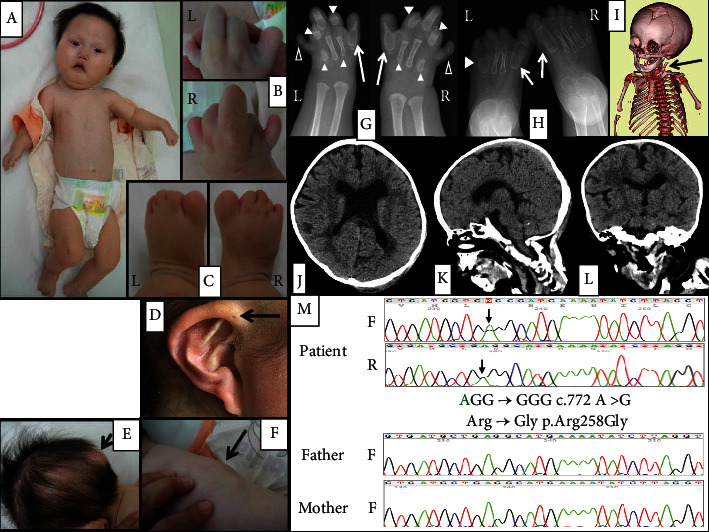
Clinical features, radiological examination findings, and genetic analysis results of our patient. (a–f) Clinical features of our patient at the first visit to our hospital. Left (L); right (R). (a) General appearance. Thin eyebrows, low-set ears, hypoplastic mandible, narrow chest, and shallow umbilicus were observed. (b) Hands. Incomplete syndactyly was observed in both hands. All fingers lacked nails. (c) Feet. Incomplete syndactyly was observed in both feet. All toes lacked nails. (d) Right ear. The lower part of the ear was hypoplastic. A periauricular sinus (arrow) was observed. (e) Flare-up observed at the occipital region of her head (arrow). The hair was sparse. (f) Flare-up observed at her back (arrow). (g–l) Results of radiological examinations. Left (L); right (R). (g) Roentgenograph of the hands at 7 months of age. Agenesis of all distal phalanges, second and fourth middle phalanges, and fifth metacarpal bone, fusion of enlarged first middle metacarpal bone and proximal phalange (arrows), shortened second and fourth metacarpal bones, and proximal phalanges (closed arrowheads), and hypoplasia of the fifth middle phalanges and metacarpal bones (open arrowheads) were observed. Osteochondromas were observed in both first fingers. (h) Roentgenograph of the feet at 2 years of age. Thick first metacarpal bones (arrows) and fused left fourth and fifth metacarpal bones were observed (closed arrowheads). (i) 3D CT analysis at 7 months of age. The left sternocleidomastoid muscle was ossified (arrow). Craniosynostosis was not observed. (j–l) Head CT analysis at 6 years of age. (j) Horizontal view. The septum pellucidum was lacking. The lateral ventricle was enlarged. (k) Sagittal view. The corpus callosum was thin, and a deformed brain stem with bulging dorsal pons was observed. (l) Coronal view. Not only the lateral ventricle but also the third ventricle was enlarged. The sylvian fissure was wide. (m) Results of the genetic analysis of the patient and her parents. A heterozygous base substitution from adenine to guanine at position 772, resulting in an amino acid change from arginine to glycine at 258, was observed in the patient (arrow). This substitution was not observed in either of her parents. Forward strand (F); reverse strand (R).
